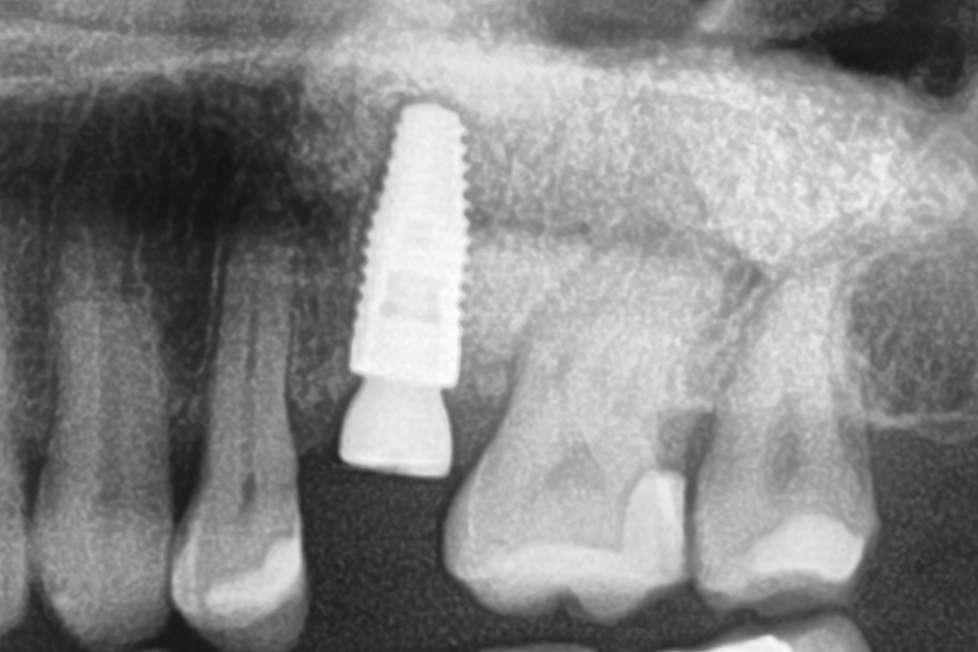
Radiograph after a subgingival healing phase of eight months and insertion of a transgingival abutment
